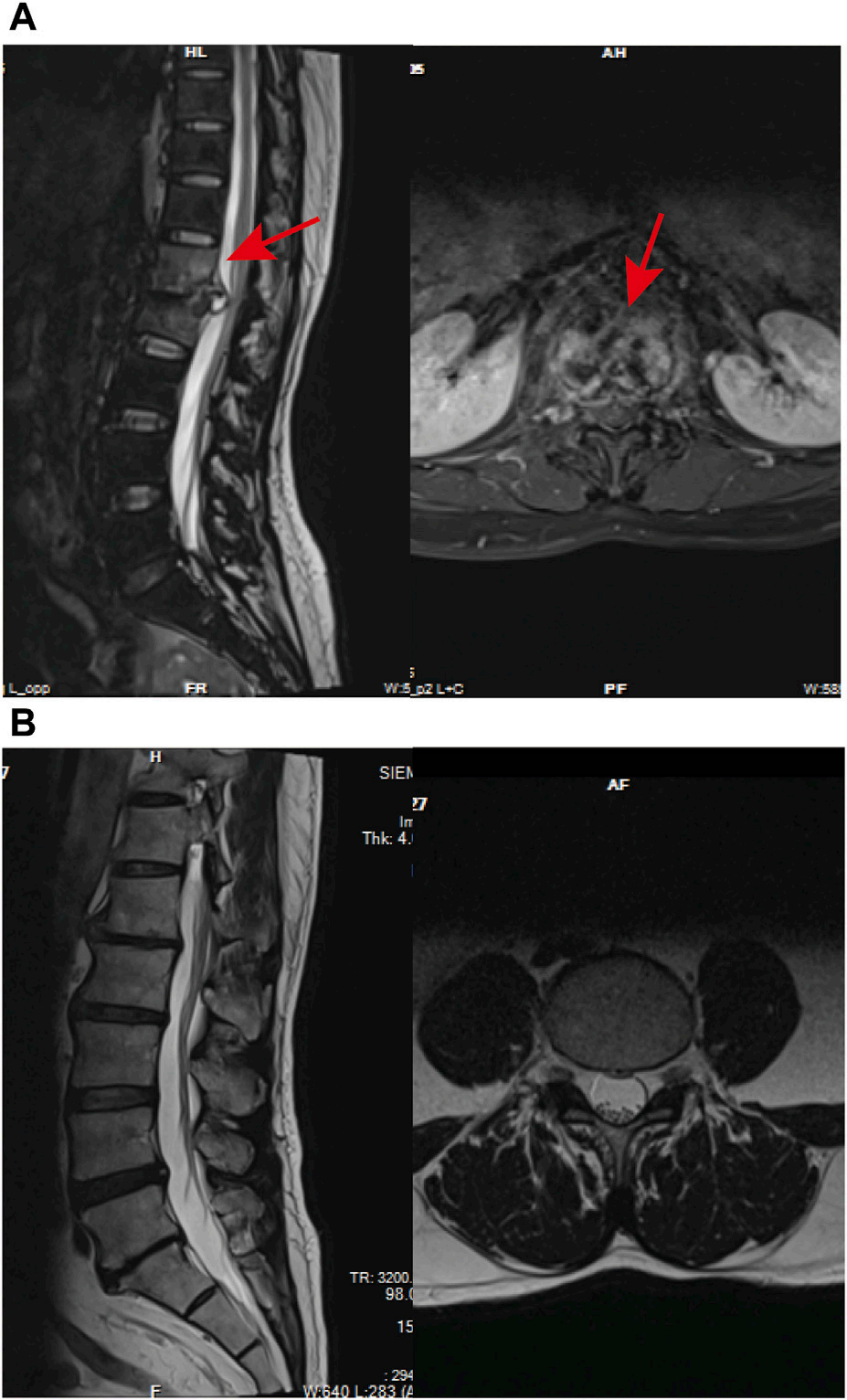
Radiographic manifestations of spinal tuberculosis. (A) Radiographic manifestations of spinal tuberculosis (indicated by arrows). (B) Imaging features of a normal spine.
